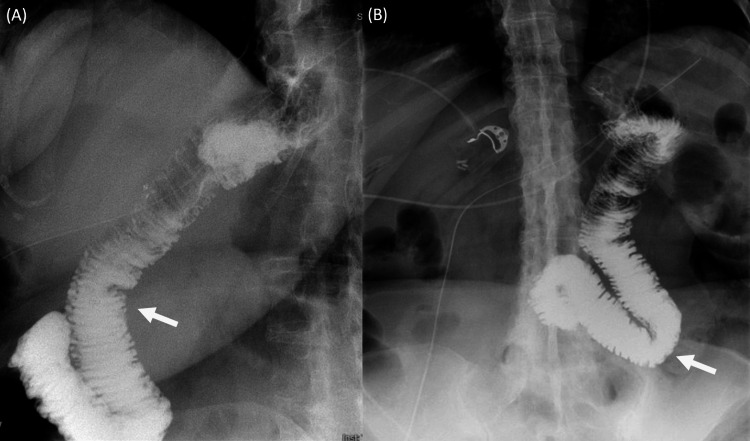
Post-gastrectomy upper GI X-ray series with barium swallow shows anastomosed bowel without evidence of a leak. Panel A corresponds to the lateral X-ray. Panel B corresponds to the anterior-posterior X-ray. The white arrows indicate barium.
